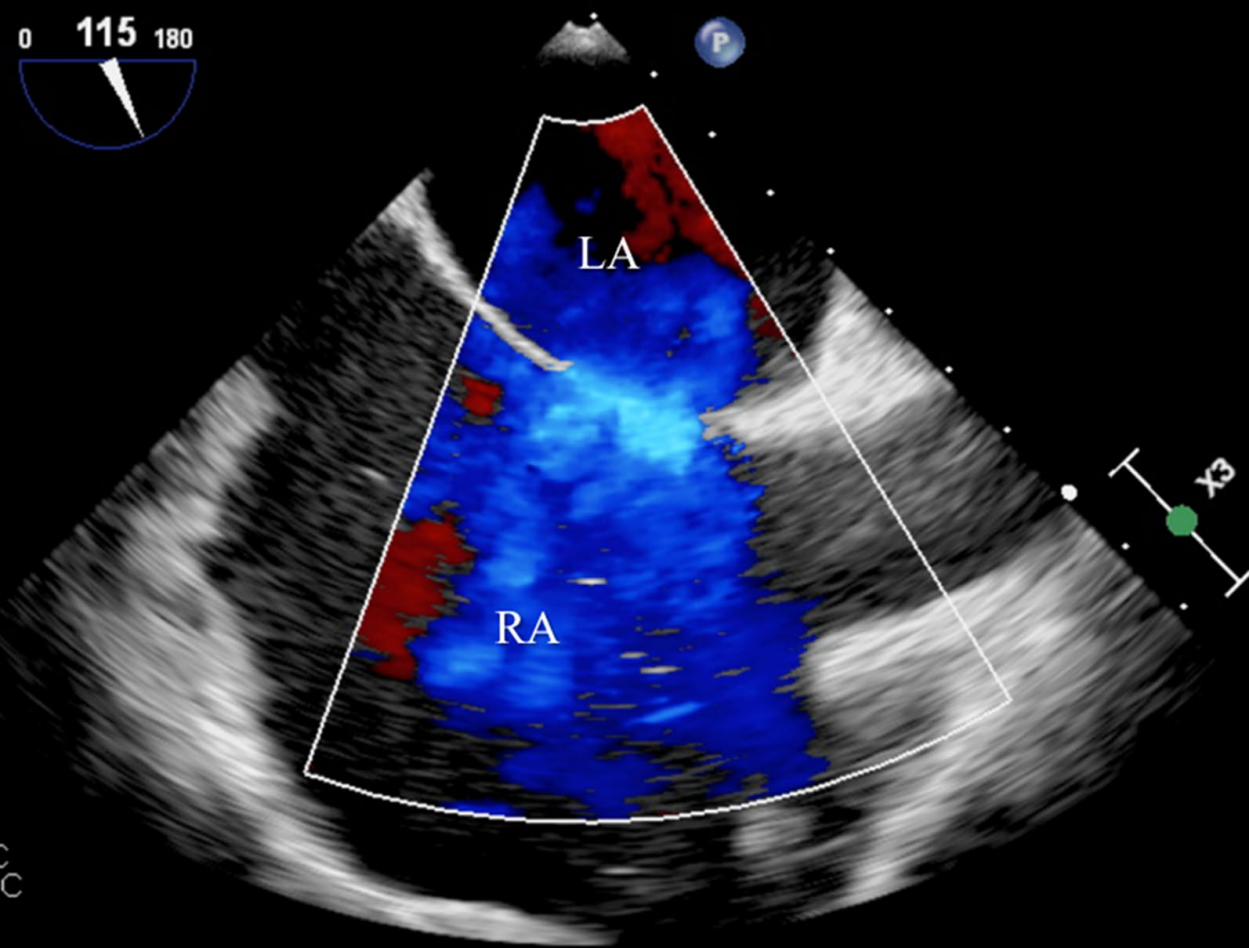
Color doppler imaging showing a secundum ASD with left-to-right shunt on TEE. *ASD* atrial septal defect, *LA* left atrium, *RA* right atrium, *TEE* trans-esophageal echocardiography
